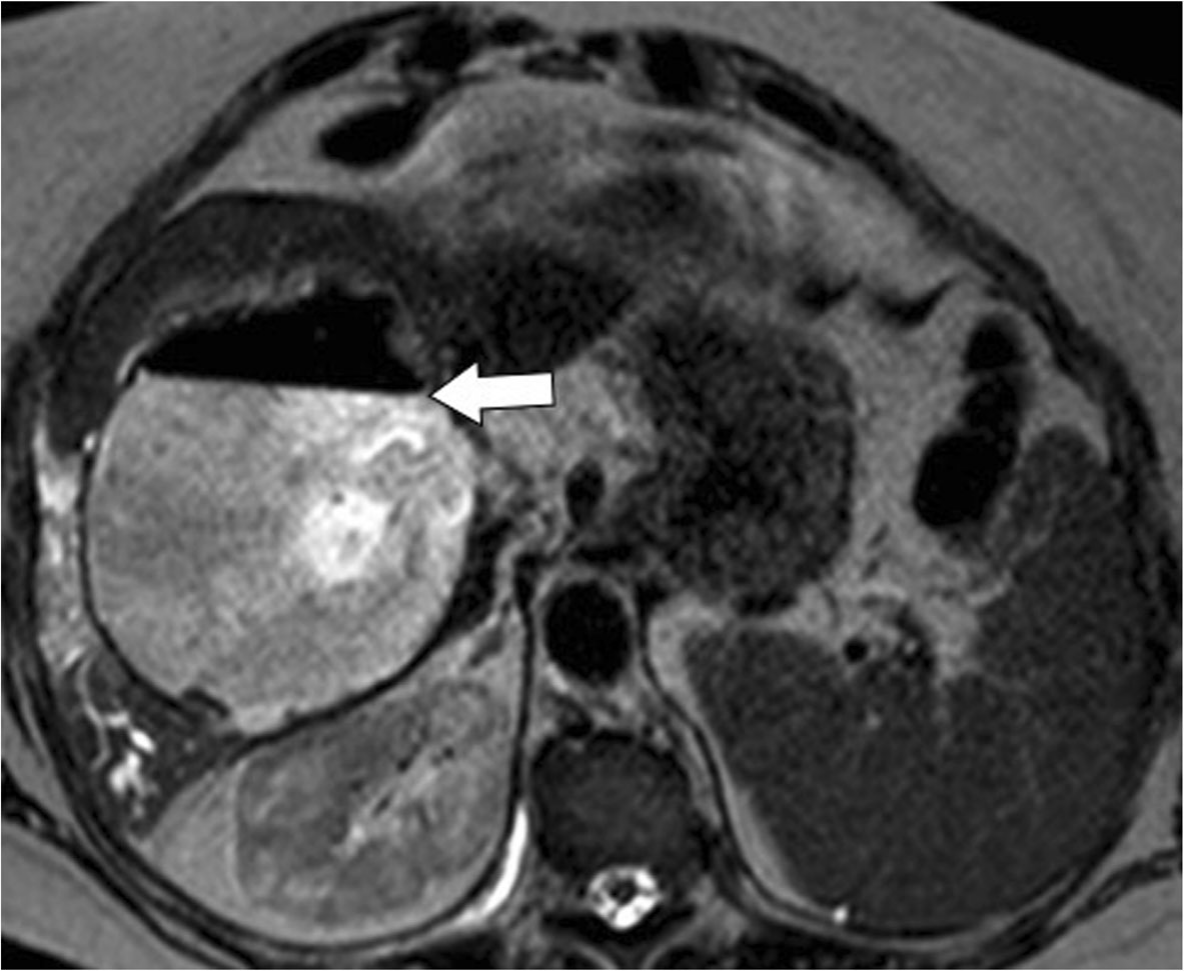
A 66-year-old woman with infected hydatid cyst. Axial T2-weighted MR image demonstrates an air-fluid level (arrow) within a calcified hydatid cyst, but lack of frank cysto-biliary communication. This finding suggests superinfection of a ruptured hydatid cyst that was later proved at surgery
